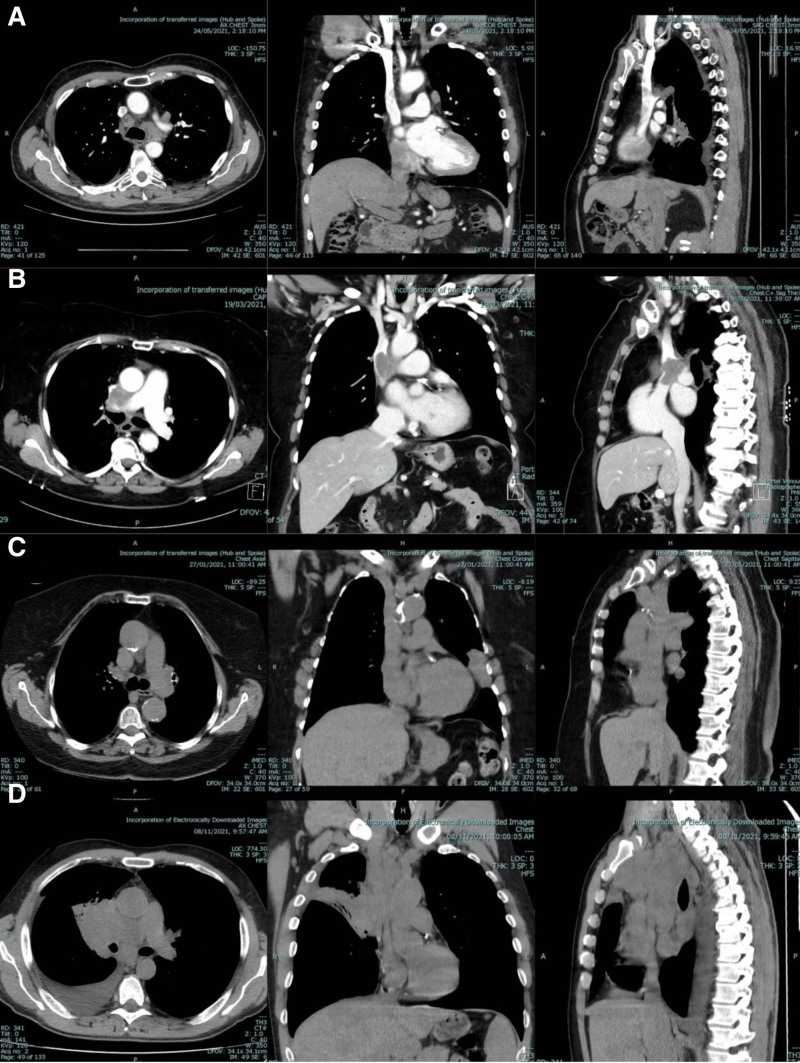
Examples of the presence and absence of SVCO in CT imaging with and without contrast. (A) Example of contrast-enhanced CT chest without obstruction; (B) example of contrast-enhanced CT chest with obstruction; (C) example of non-contrast CT chest without obstruction; (D) example of non-contrast CT chest with likely obstruction. CT = computed tomography, SVCO = superior vena cava obstruction.
